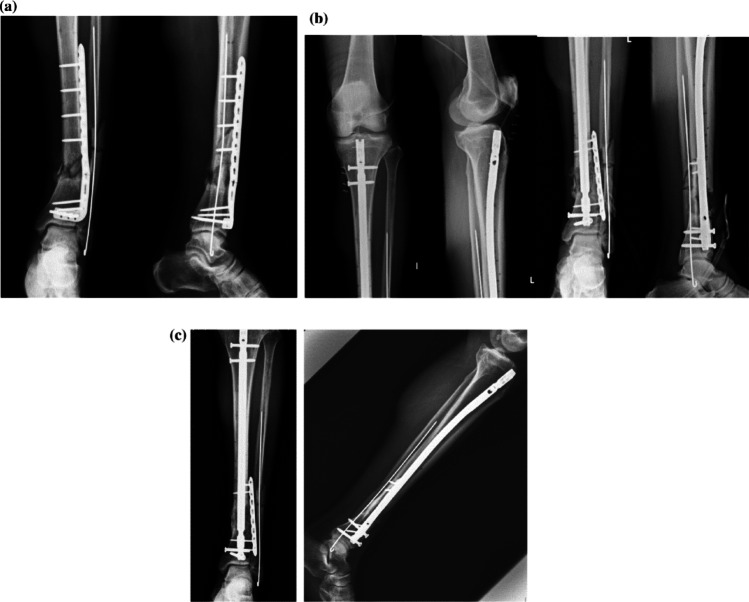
Representative case of a 70-year-old man after a pedestrian injury. a Four months after plate fixation for the distal tibial fracture, radiographs demonstrate fracture nonunion and implant failure. b Post-operative radiographs after the patient was operated through a nail-plate construct. c Nine months after the revision surgery, bone union is noted and the patient could walk freely without support
